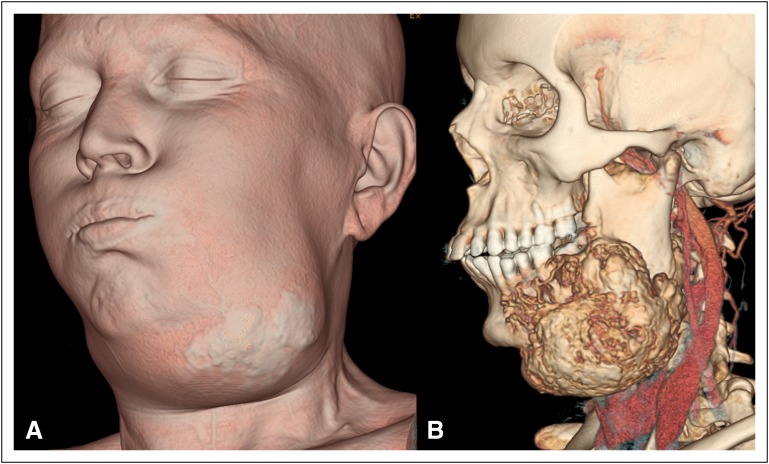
(A and B) Three-dimensional volume-rendered computed tomography images showing the bone beard tumor along the mandible.
